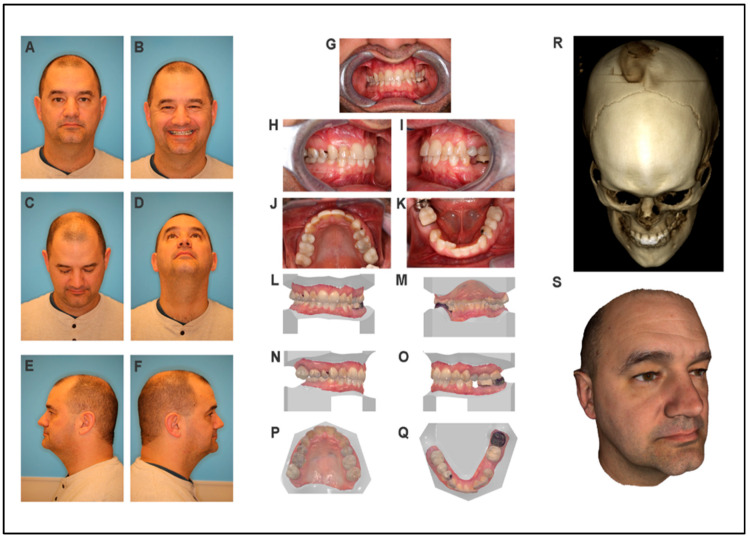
Clinical assessment of the unaffected father of the proband. (A–F) Two-dimensional photographs of the proband’s father. (G–K) Intraoral photographs (oral photo is flipped). (L–Q) Intraoral scan images. (R) Three-dimensional CBCT scan image. (S) Three-dimensional soft tissue image.
